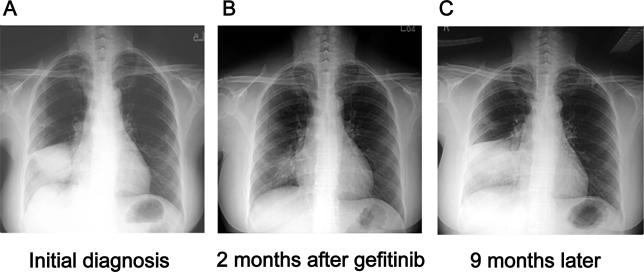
Chest Radiography Chest radiography shows a large mass in the RLL before gefitinib treatment (A), and marked decrease in tumor size two months after gefitinib was initiated (B). This tumor progressed nine months after gefitinib treatment (C).
